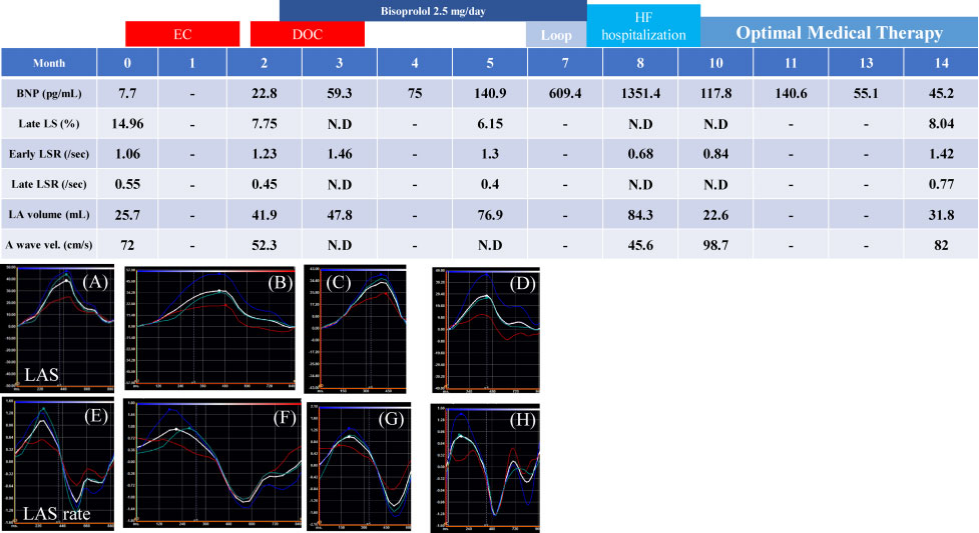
Clinical timeline describing the patient’s clinical presentation, including plasma brain natriuretic peptide levels and transthoracic echocardiography findings, and the treatment approach (upper panel). Lower figures demonstrate the left atrium waveform obtained by the two-dimensional speckle tracking method. (*A–D*) Left atrium strain and (*E–H*) left atrium strain rate. (*A* and *E*) Evaluation before cancer treatment, (*B* and *F*) 2 months after the initiation of cancer treatment, (*C* and *G*) 3 months after the initiation of cancer treatment, (*D* and *H*) 5 months after the initiation of cancer treatment. DOC, docetaxel administration; EC, epirubicin and cyclophosphamide administration; HF, heart failure; LA, left atrium; LAS, left atrium strain; Loop, loop diuretic (daily oral 10 mg furosemide administration); LS, longitudinal strain; LSR, longitudinal strain rate.
